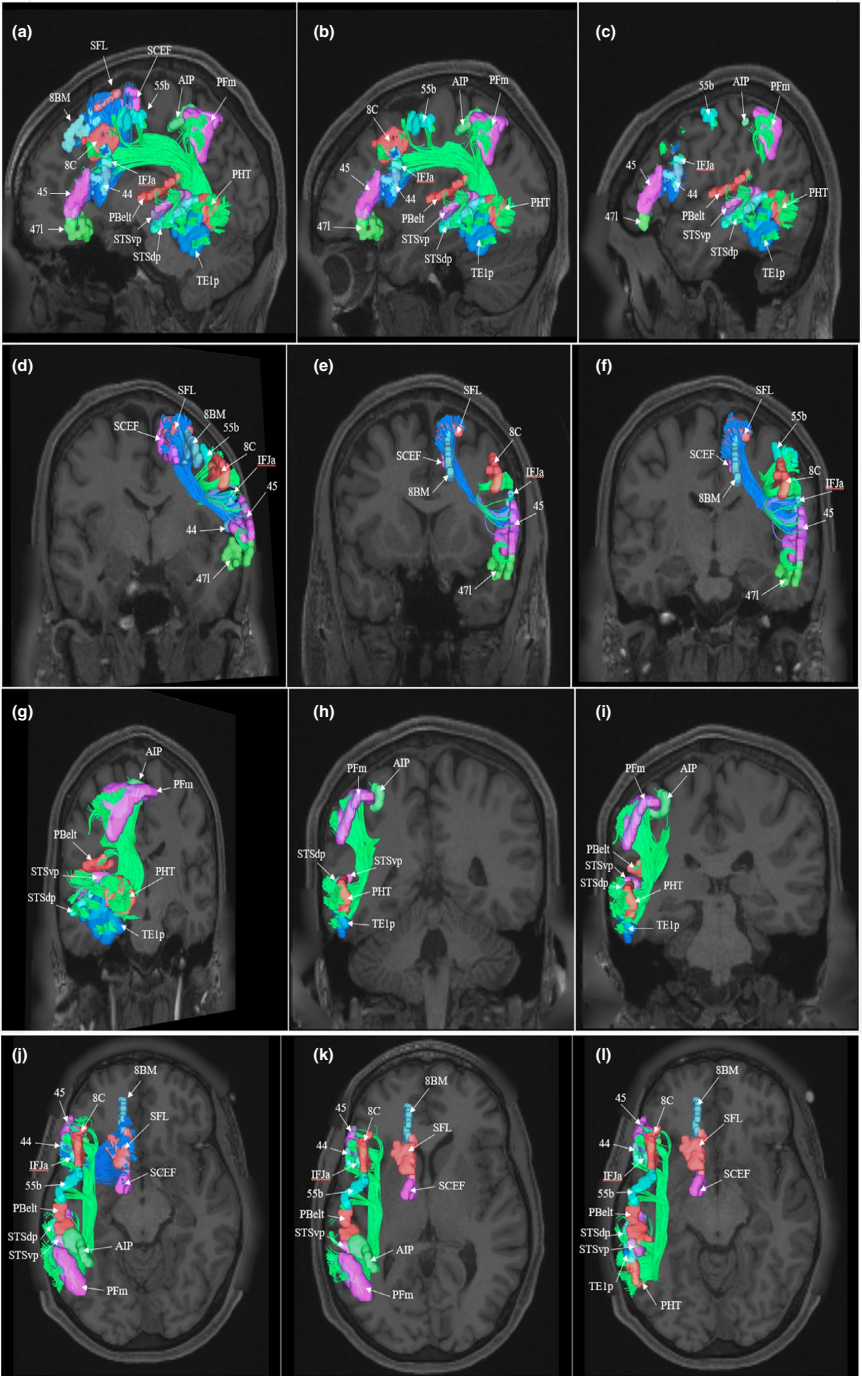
Fiber tracking analysis for the semantic network. Shown on T1‐weighted MR images in the left cerebral hemisphere. TOP ROW: sagittal sections from most medial to most lateral demonstrating the superior longitudinal fasciculus and its projections between the frontal, parietal, and temporal clusters of the dorsal attention network. ROW TWO AND THREE: Partially oblique (left column) and pure (middle and right column) coronal sections. BOTTOM ROW: axial sections through the frontal and parietal clusters of the network. The fronto‐parietal projections of the SLF are particularly apparent
